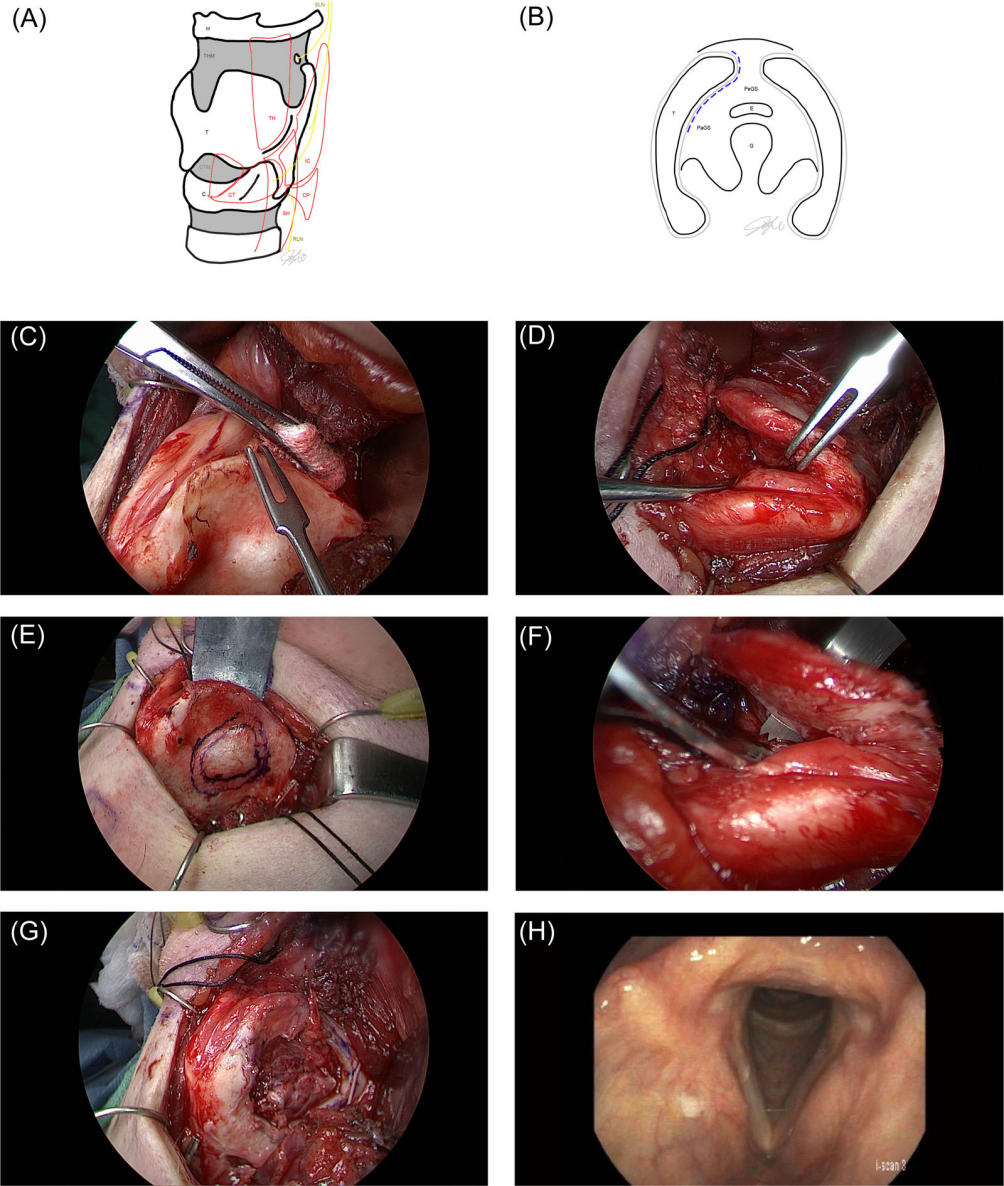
Laryngeal framework with relevant anatomy. (A) Oblique and (B) axial views at the level of the thyroid ala. The dotted line demonstrates dissection in the paraglottic space. Intraoperative (C) oblique and (D) superior views of the paraglottic space, with (E) marking and (F) resection of the neoplasm and (G) the final defect displayed. (H) Flexible laryngoscopy at 10 weeks postoperatively. C, cricoid cartilage; CP, cricopharyngeus muscle; CT, cricothyroid muscle; CTM, cricothyroid membrane; E, epiglottis; G, glottis; H, hyoid; IC, inferior constrictor muscle; PaGS, paraglottic space; PeGS, pre‐epiglottic space; RLN, recurrent laryngeal nerve; SLN, superior laryngeal nerve; ST, sternohyoid muscle; T, thyroid cartilage; TH, thyrohyoid muscle; THM, thyrohyoid membrane.
